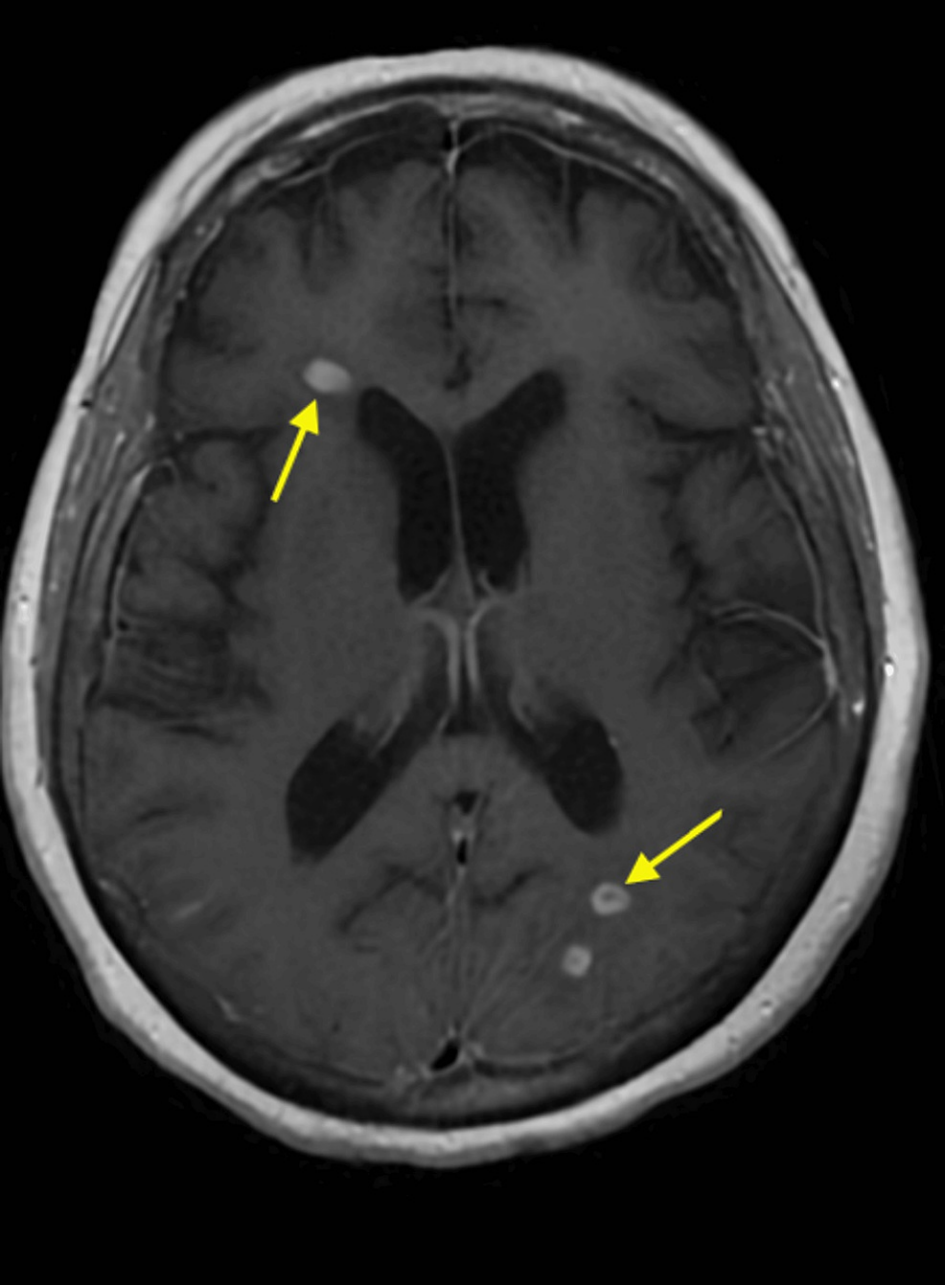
Magnetic resonance imaging (MRI) showing multiple brain abscesses
